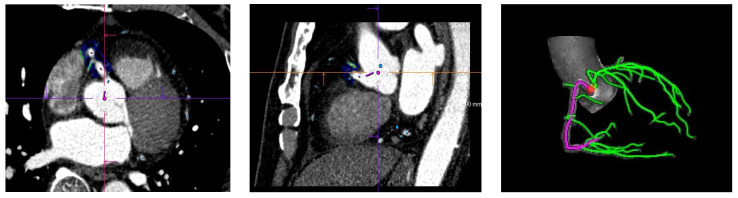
Coronary artery reconstruction: CTA data were imported into the Siemens research prototype FAI analysis software (CT Coronary Plaque Analysis, software version 5.0.2, Siemens-healthineers GmbH; Forchheim, Germany), which automatically performed 3D reconstruction of the LAD, LCX, and RCA.
